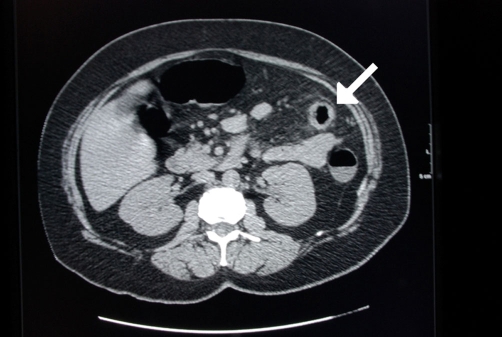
Abdominal CT showing a stricture of the sigmoid colon in ulcerative colitis (arrow).
